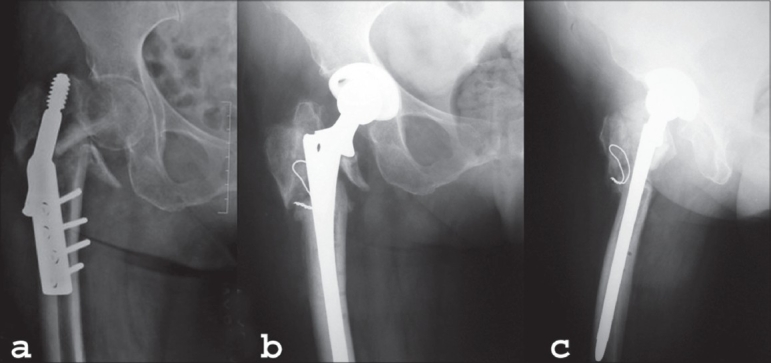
(a) Preoperative X-Ray (AP view) of right hip shows failed DHS for intertrochanteric fracture femur. (b) Postoperative X-Ray (AP and lateral view) of the same hip at 15 months of cementless revision stem (‘Solution’™)
